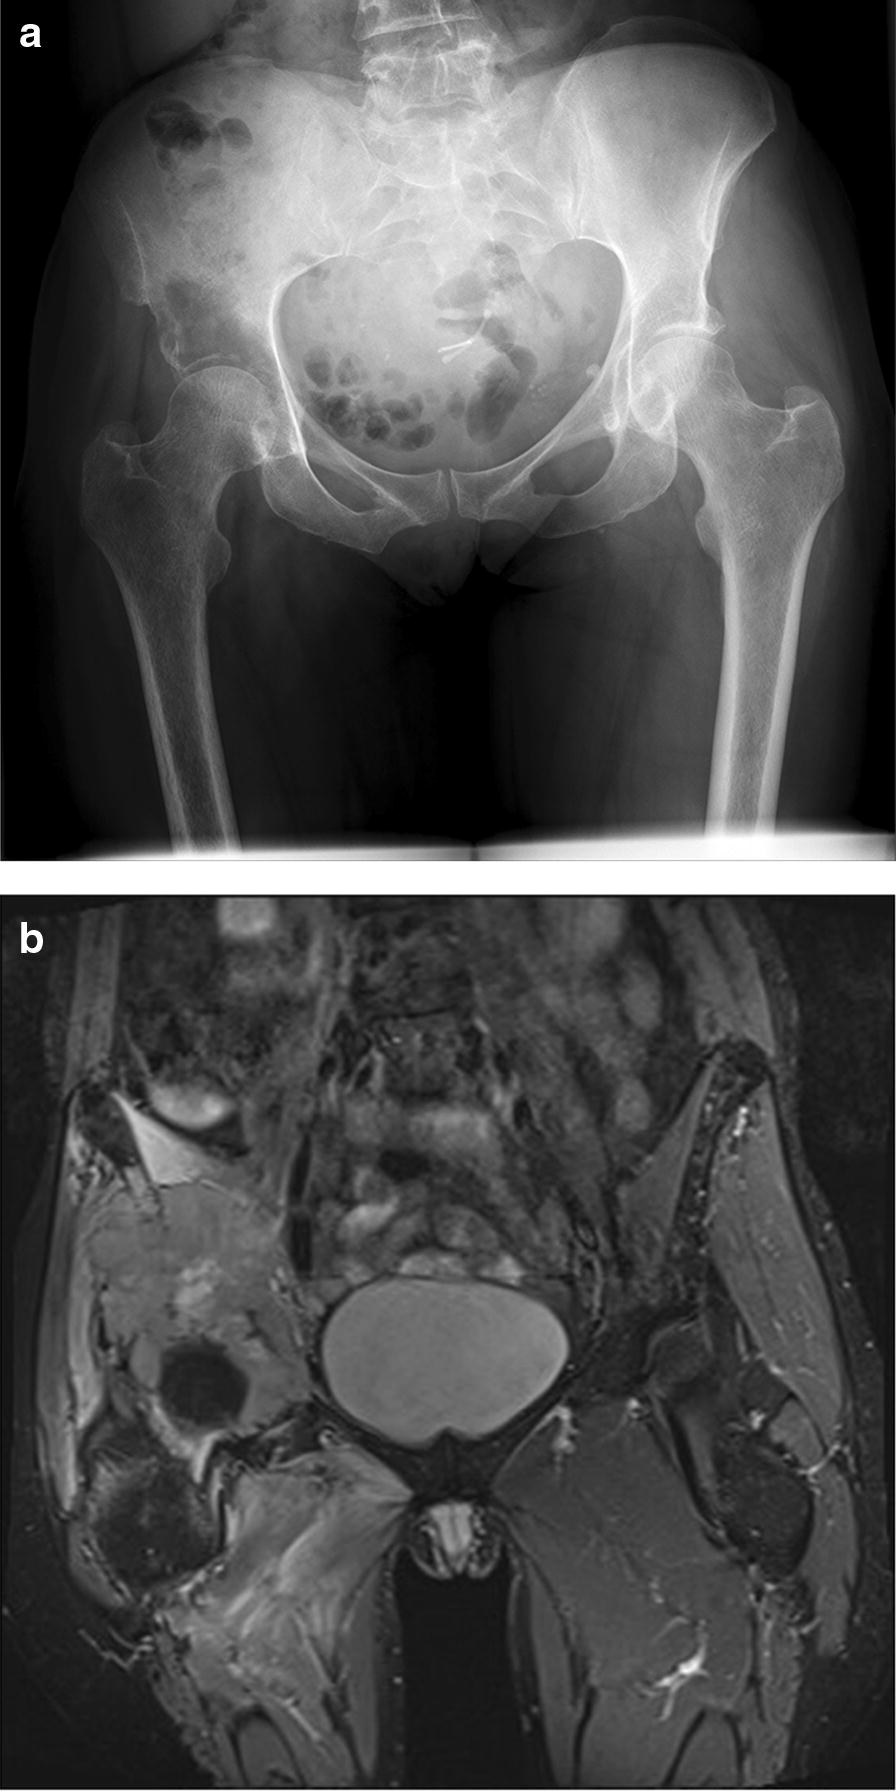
Pre-operative pelvis radiograph (a) and MRI (b) of a patient who underwent HQA. In this case, there was a large tumour involving both the hip joint and femoral neurovascular bundle
